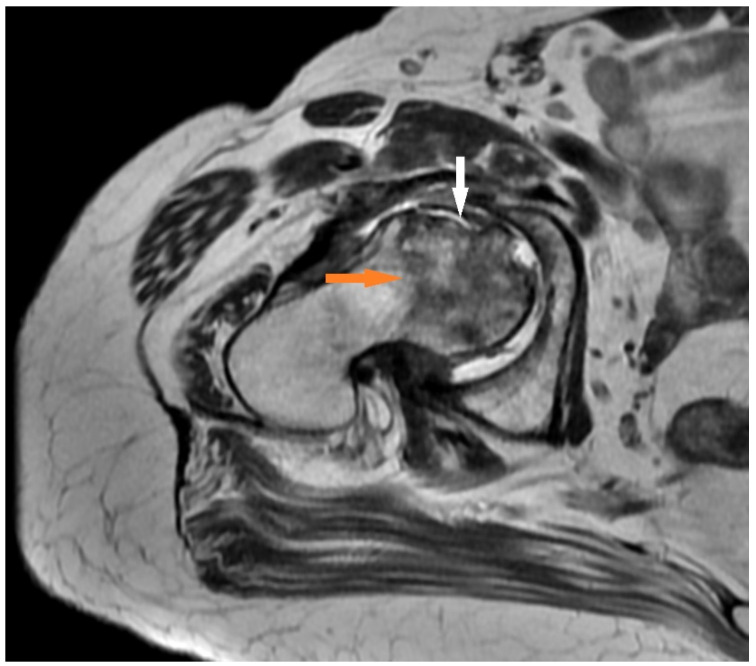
Changes found in a T2-weighted sequence in a 62-year-old man: delineation of the necrosis towards the femoral neck (orange arrow) and destruction of the anterior femoral head with impression of the cortical bone (white arrow).
